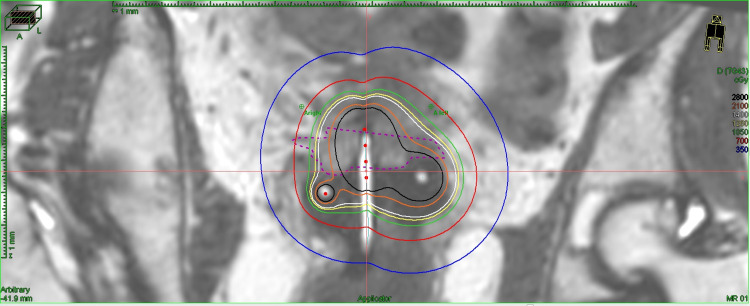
Dose profile for the first fraction on MRI using an IC approach alone MRI: magnetic resonance imaging; IC: intracavitary
